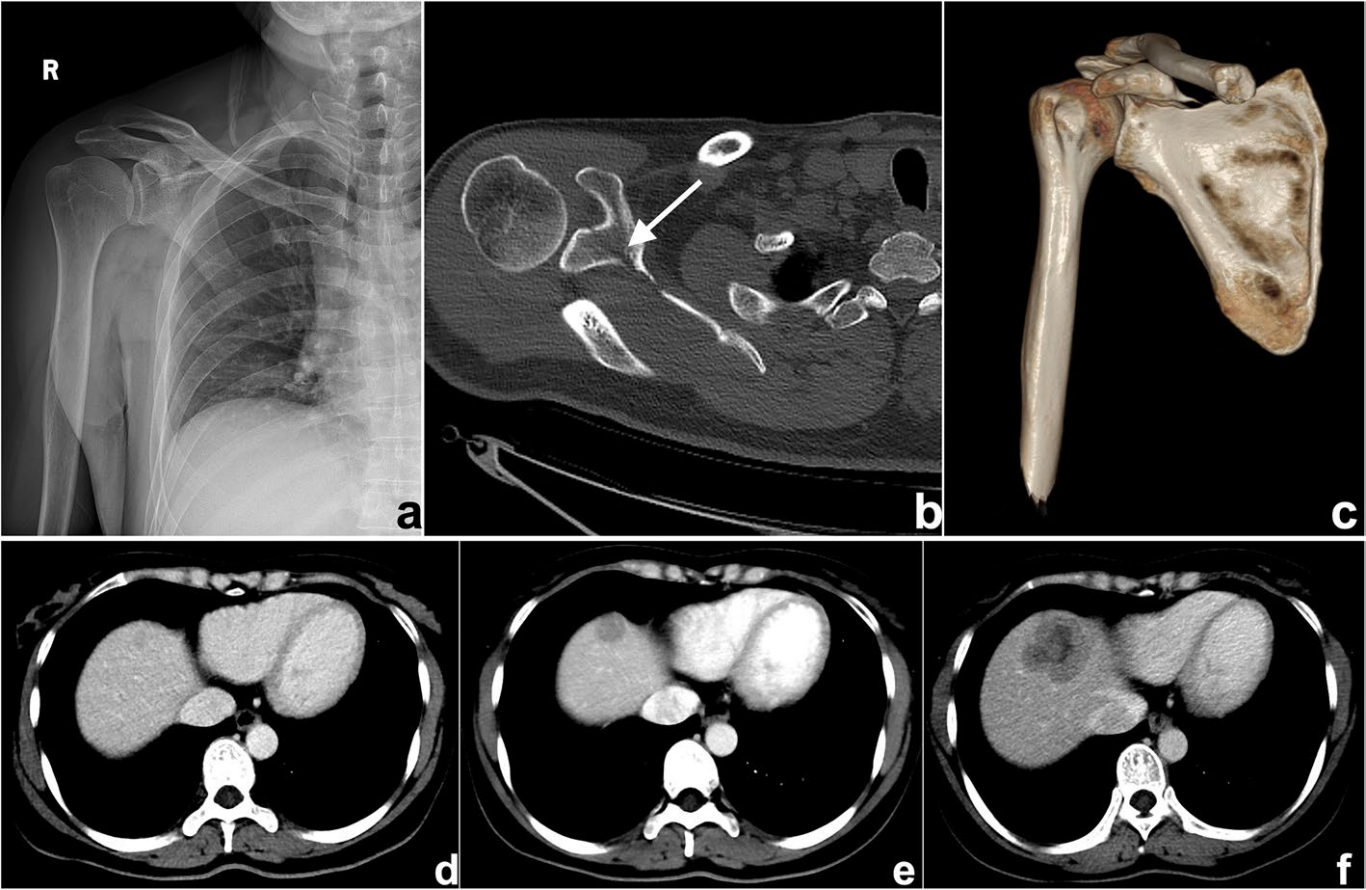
a–c A 47-year-old man suffering from right shoulder pain for 3 days after trauma. a Plain radiography was reported to be normal because the radiologist missed the subtle low-density fracture line due to the overlapping position and careless reading. Axial computed tomography (CT) image (b) and three-dimensional (3D) reconstruction (c) showed scapular fractures below the coracoid (arrow). d–f Gradually enlarged low-density liver metastasis in a 32-year-old woman after radical resection and chemotherapy of sigmoid cancer. The images demonstrated an ill-defined margin and heterogeneous enhancement. The lesion was not detected and reported in a timely manner from the first two CT scans (d, e) because it was small and located at the top of the liver
